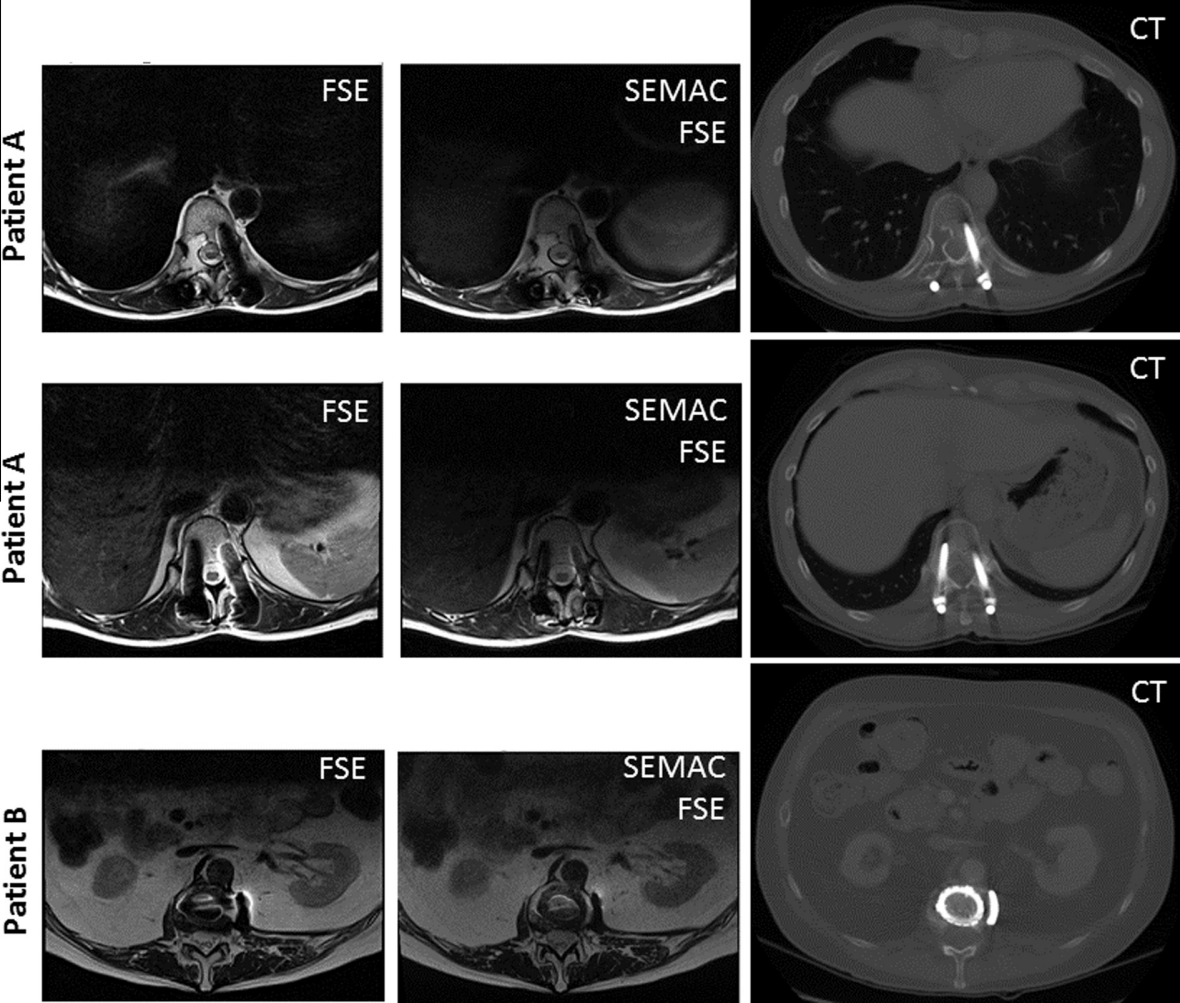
Spine images where the spinal canal was at least partially obscured by artifacts with FSE techniques: two slices on patient A and one on patient B. Areas of signal loss and signal pile up are visible with conventional FSE techniques, but minimised with SEMAC FSE techniques, enabling better registration between MRI and CT and complete visualisation of the spinal cord within the spinal canal.
